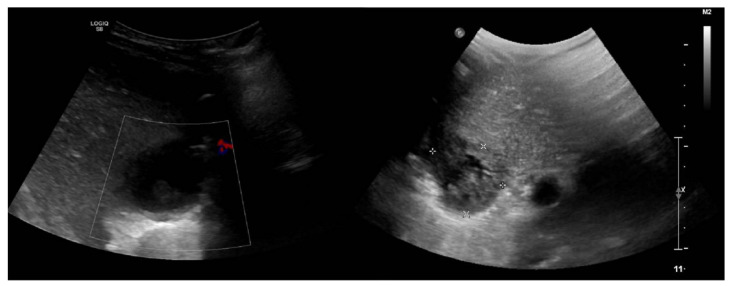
Abdominal ultrasound. Hepatic hypoechoic lesion (approximately 3.5 × 3 cm) with well-defined margins (6 mm) located in the right lobe.
